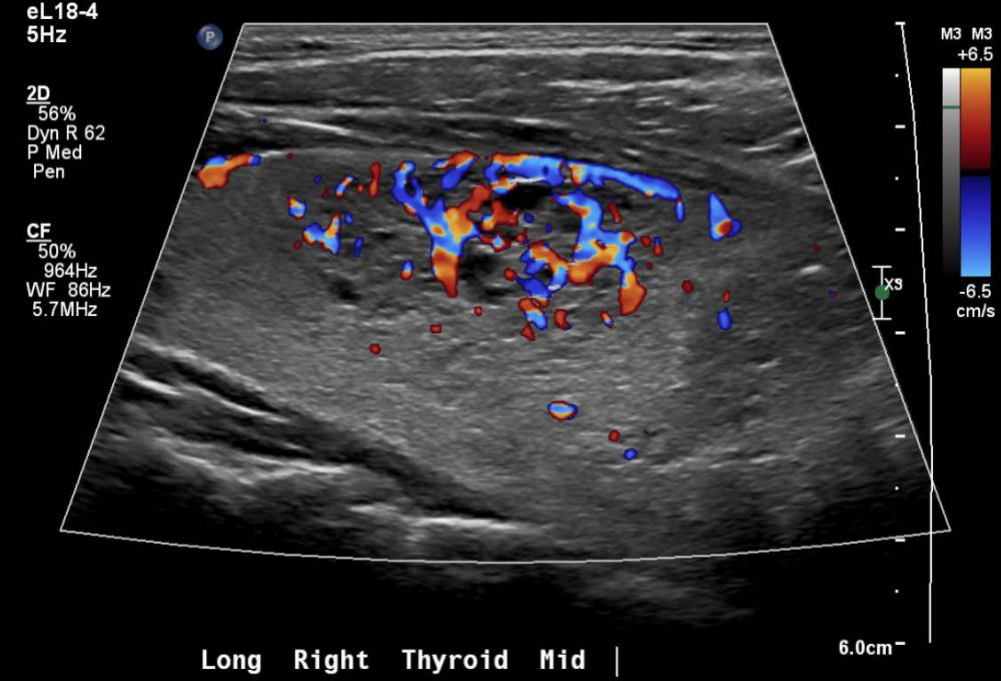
Longitudinal: right medial mid gland with Doppler.
